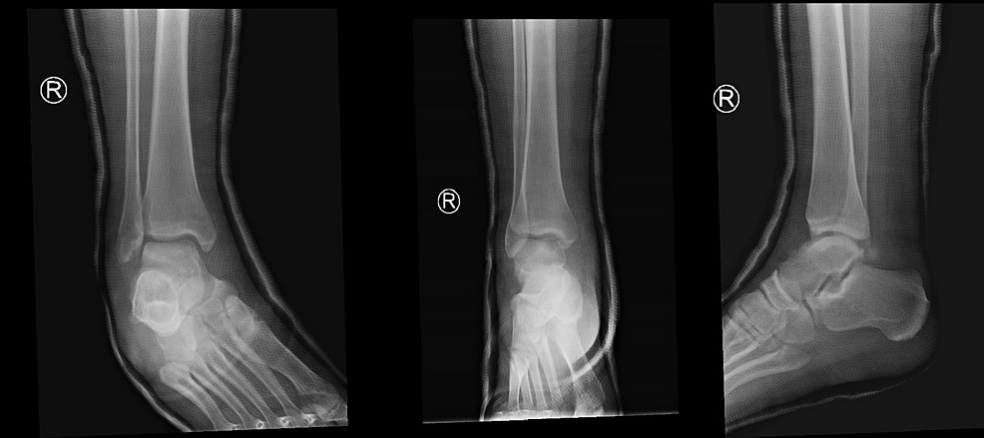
Plain X-rays at six-week follow-up.
